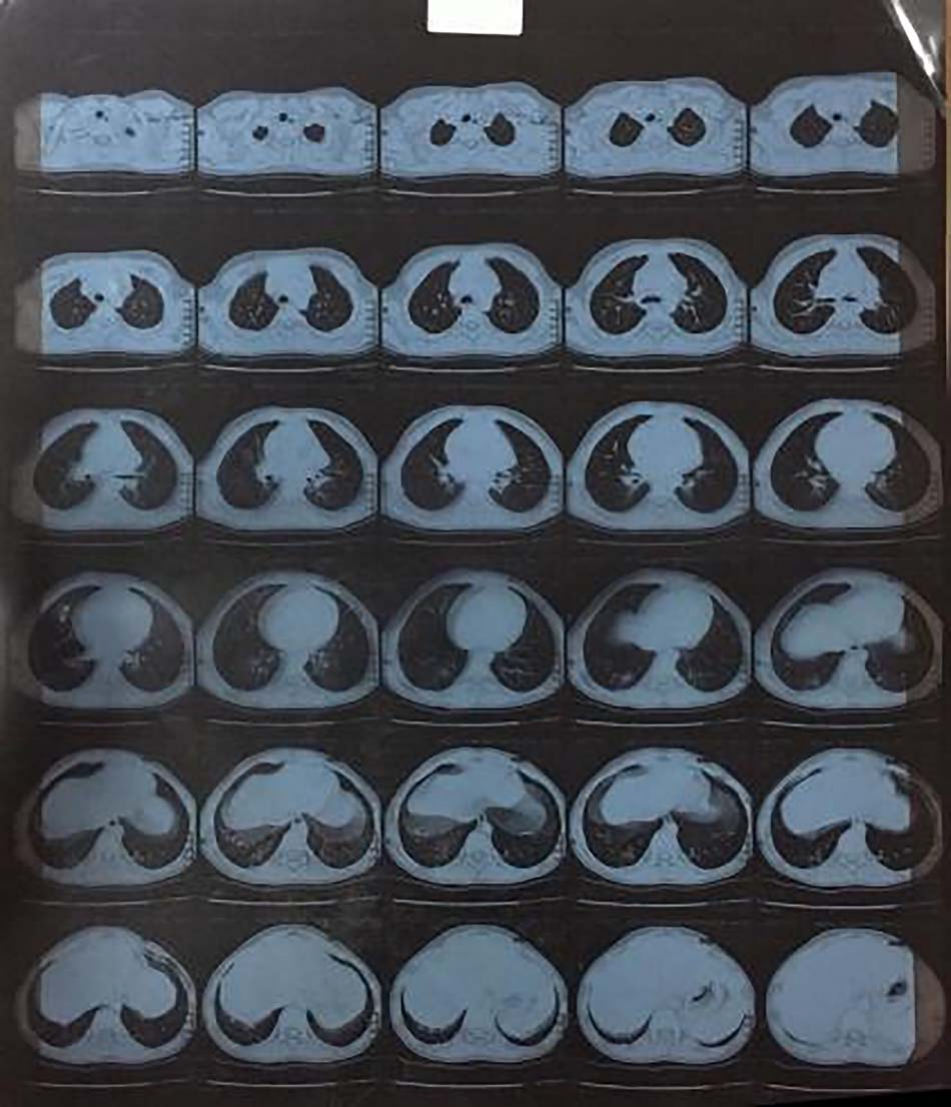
CT showing homogeneously hypo-echoic thymic mass seen in the retroperitoneal region at the level of the right hilum with enlarged lymph nodes.
